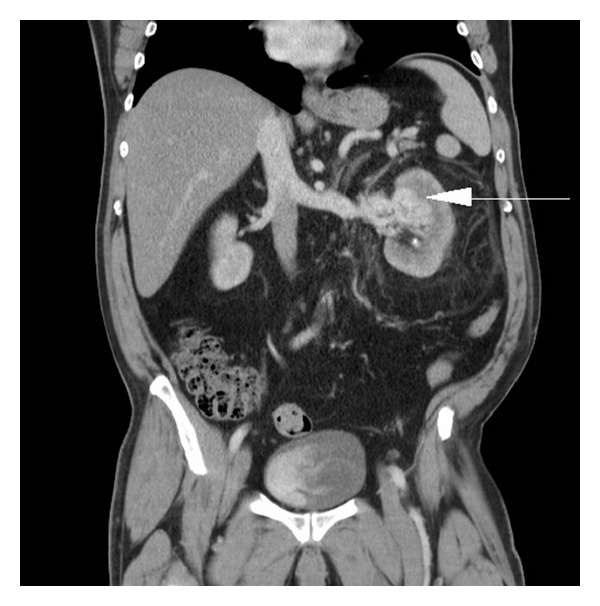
Coronal CT image showing a vascular tangle (arrow) in the left kidney.
